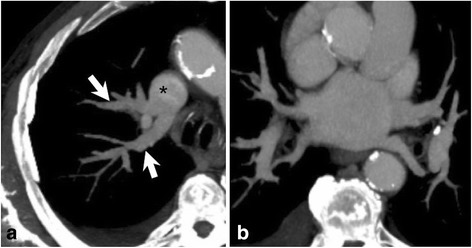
Contrast enhanced CT demonstrate anomalous venous drainage with two pulmonary veins (a, arrows) draining the right upper lobe into the superior vena cava (a, asterisk). Pulmonary veins are normal (b). Findings are consistent with partial anomalous pulmonary venous connection
